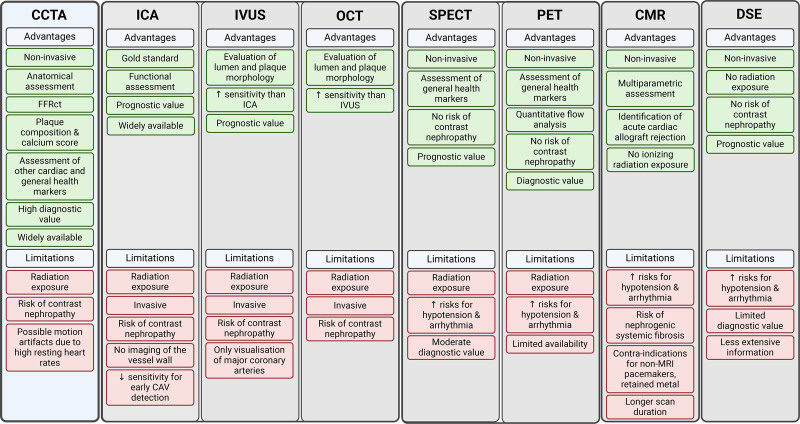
Comparison of CCTA to other imaging modalities. CCTA, coronary computed tomography angiography; CMR, cardiovascular magnetic resonance; DSE, dobutamine stress echocardiography; ICA, invasive coronary angiography; IVUS, intravascular ultrasound; OCT, optimal coherence tomography; PET, positron emission tomography; SPECT, single-photon emission computed tomography.
